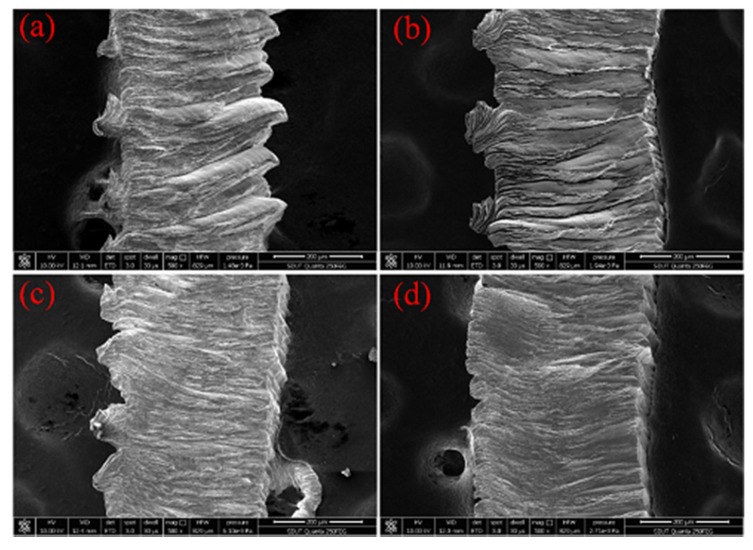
Chip morphology under (a,b) dry machining, and (c,d) ultrasonic atomization assisted machining at cutting speeds of (a,c) 20 m/min, and (b,d) 60 m/min.
